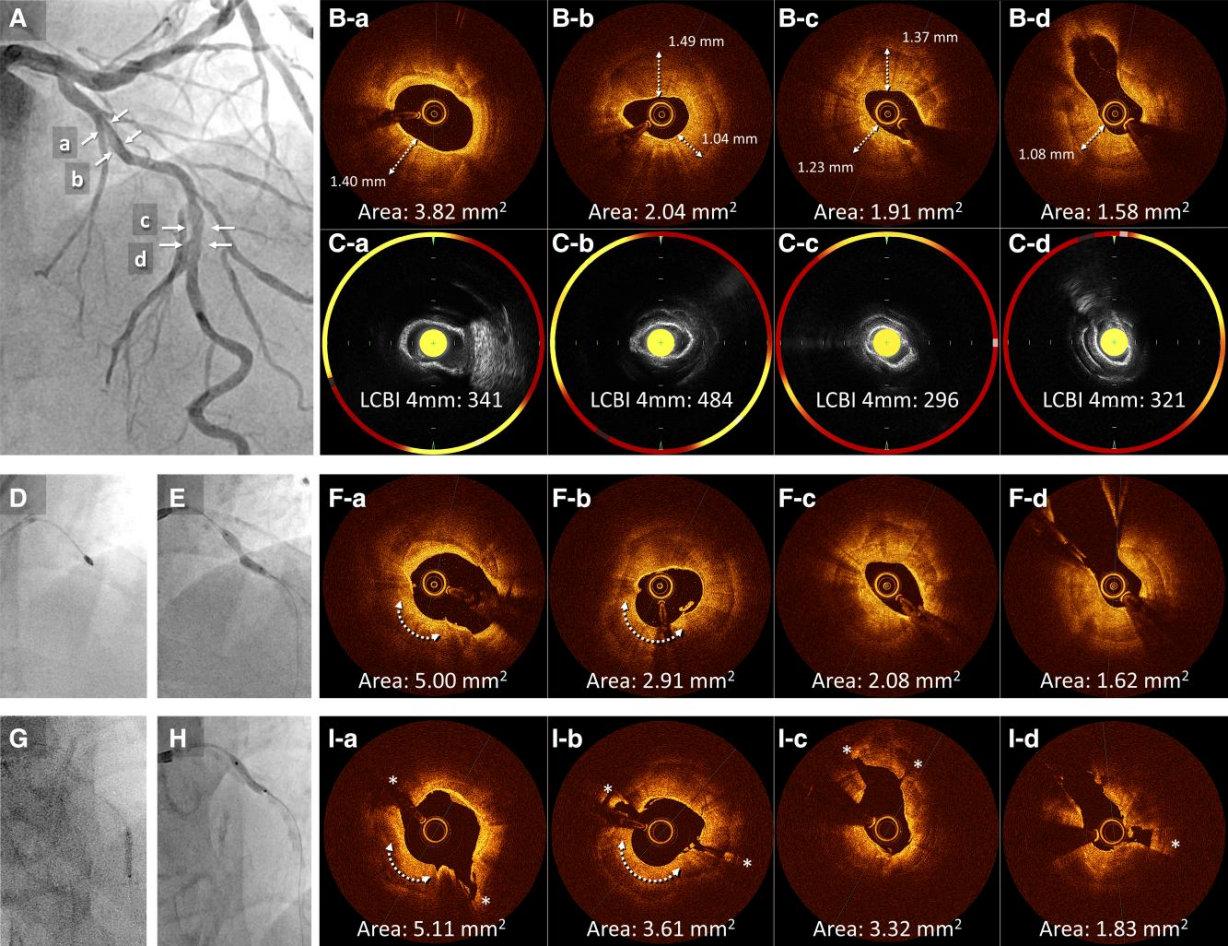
(*A*) Baseline angiography of the left anterior descending in the first session. (*B*) Baseline optical coherence tomography shows circumferential calcification with a thickness exceeding 1.0 mm in the proximal (a and b) and mid-lesions (c and d). (*C*) Near-infrared spectroscopy-intravascular ultrasound showing lipid components inside or behind the calcification (yellow areas). (*D*) Rotational atherectomy with 1.5 and 1.75 mm burrs is performed on the proximal lesion. (*E*) After Rotational atherectomy, the proximal lesion is still undilatable. (*F*) Optical coherence tomography after rotational atherectomy and balloon dilation reveals residual heavy calcification with limited lumen expansion and no calcium fractures, although the ablation effect by rotational atherectomy was observed at the 7 o’clock position of the proximal lesion (arrow). (*G* and *H*) Lithotripsy for eight cycles is performed sequentially from the mid- to the proximal left anterior descending. (*I*) Subsequent optical coherence tomography reveals multiple major disruptions (*). LAD, left anterior descending; NIRS-IVUS, near-infrared spectroscopy-intravascular ultrasound; OCT, optical coherence tomography; RA, rotational atherectomy.
